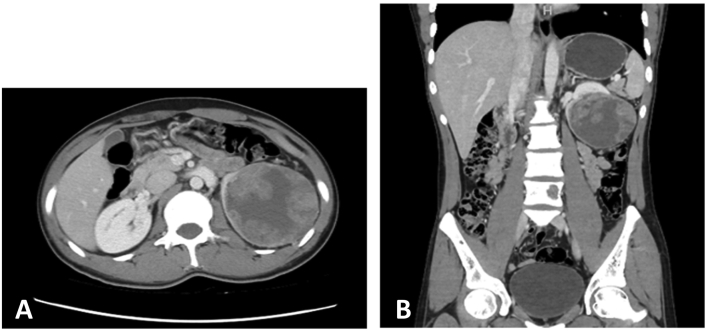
Computed tomography of the abdomen revealing a large heterogeneous left renal mass (A) axial view and (B) coronal view.
